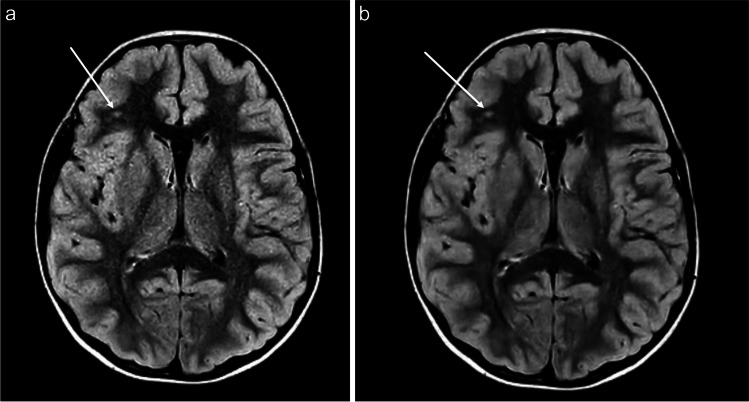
a-b Axial T2W FLAIR CS=1.6 brain MR images in an 8-year-old female presenting with repeated episodes of vomiting in the middle of the night without (a) and with (b) AI image reconstruction (SmartSpeed; Philips Healthcare). The AI image shows a reduction in image noise compared to the standard image reconstruction. There is also a focus of T2W FLAIR hyperintense signal in the right frontal white matter (arrows), doubtful clinical significance however is clearly reproduced on the AI reformat. Note is also made of a cavum septum pellucidum, normal anatomic variant, also accurately reproduced
